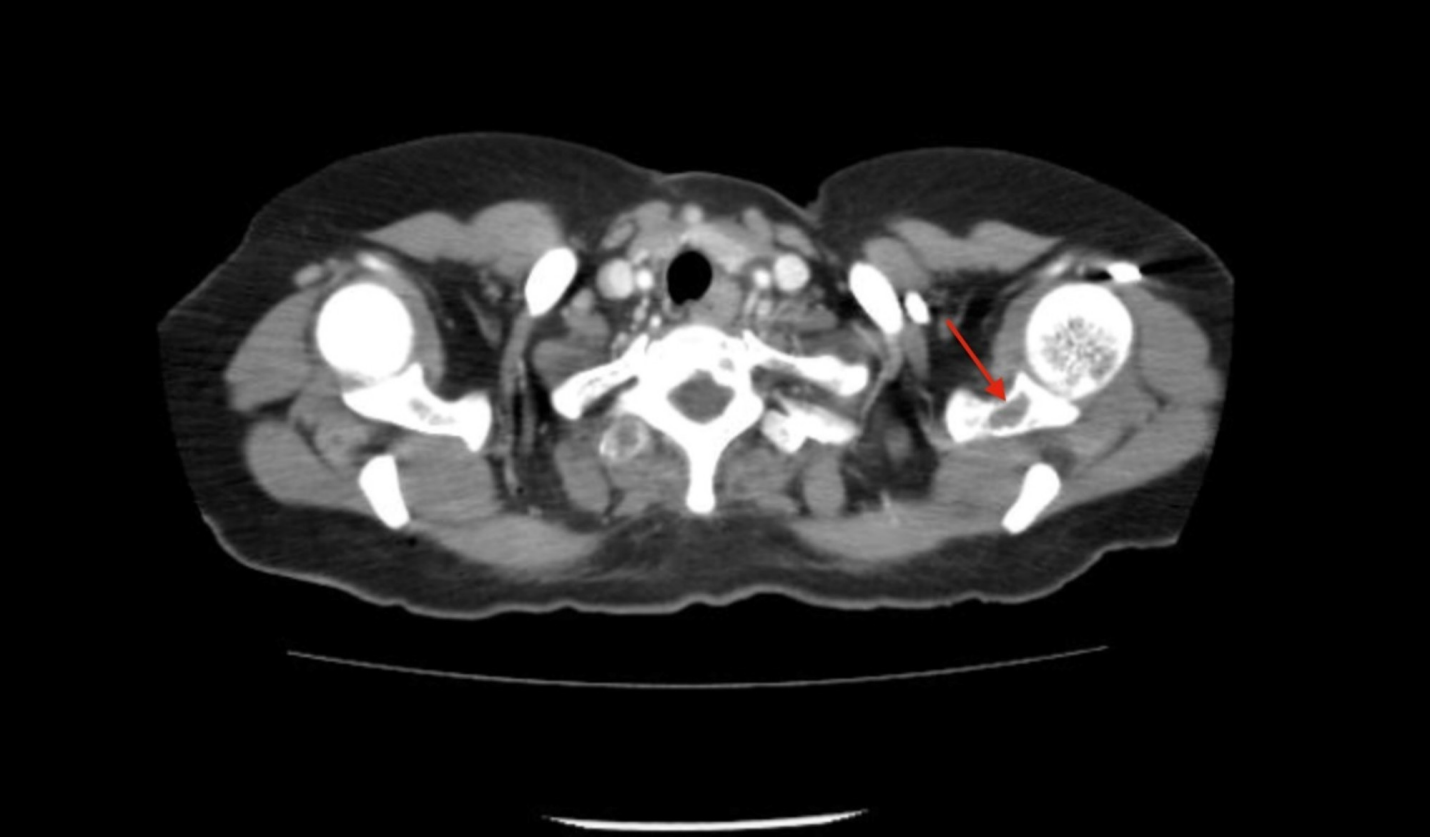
Axial section of a CT scan showing a lytic lesion in the left scapula (red arrow) CT: computed tomography
